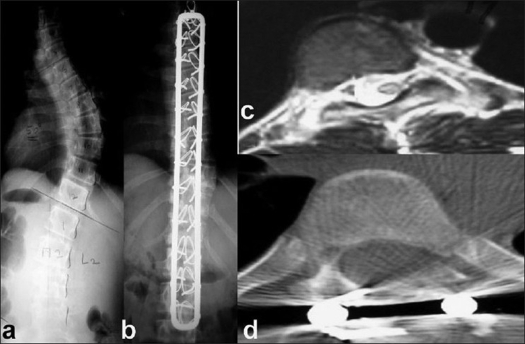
(a) X-ray dorso lumbar spine antero posterior view showing idiopathic scoliotic deformity (b) X-ray dorso lumbar spine antero posterior view showing good coronal correction of an idiopathic scoliotic deformity at five years post operative (c) MRI T2WI Axial shows rotation of the vertebral body (Pre operative) (d) CT Scan Axial image shows appreciable derotation post operatively
